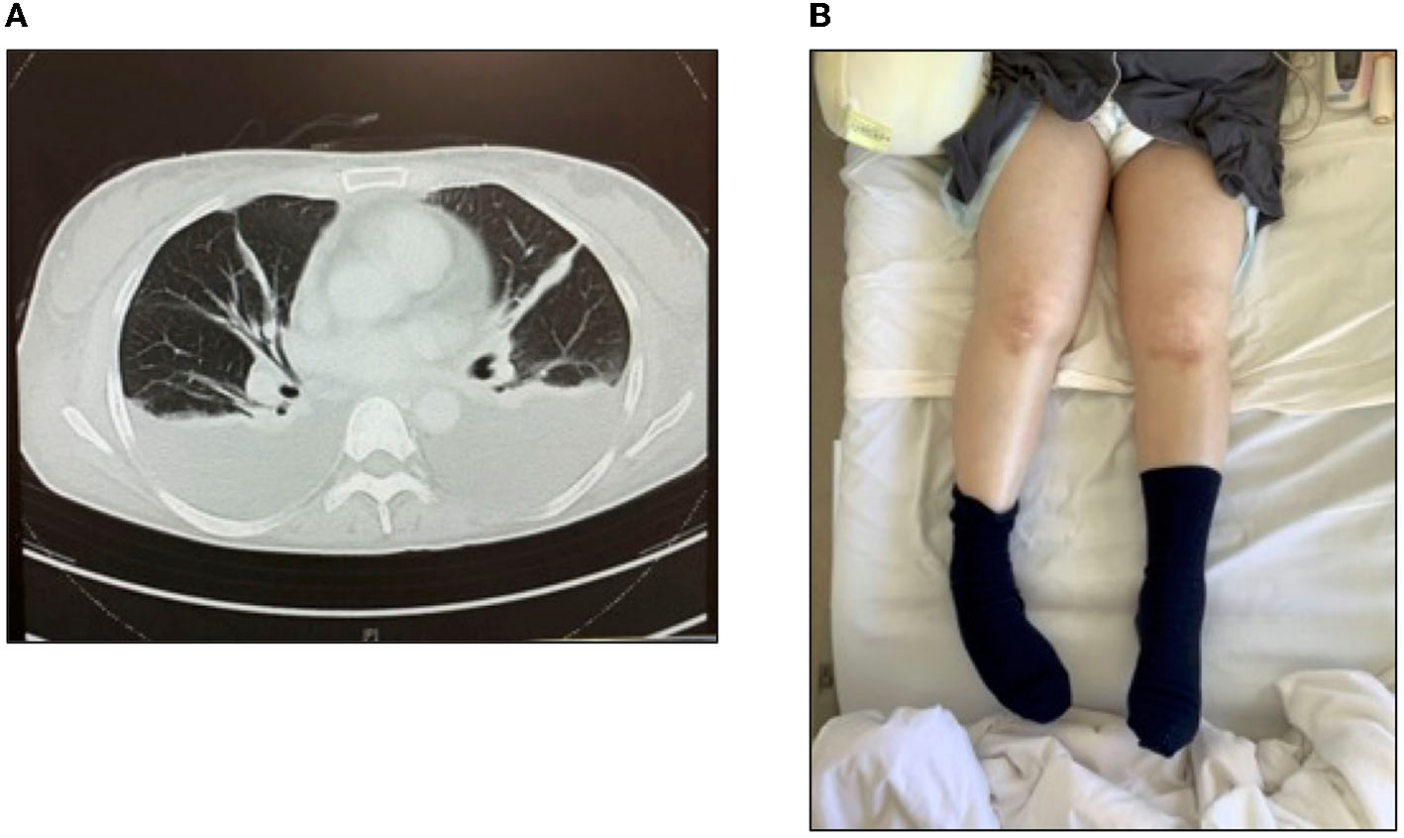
Thoracic ascites and generalized edema and the diagnostic criteria for TAFRO syndrome. (A) Computed tomography image of the chest and (B) generalized edema.
